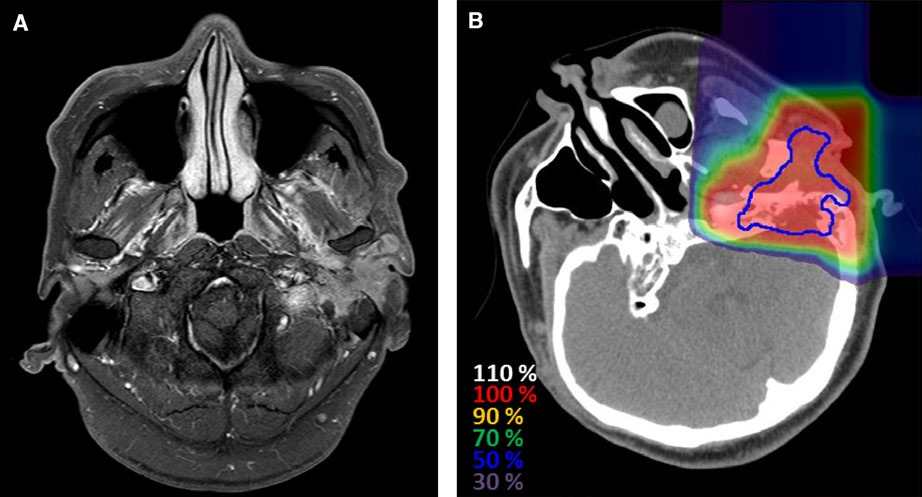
Representative case of a patient with squamous cell carcinoma of the left middle ear who was treated with carbon‐ion radiotherapy. A, Magnetic resonance imaging before carbon‐ion radiotherapy reveals a well‐enhanced left middle ear tumor B, Dose distribution. Blue lines show gross tumor volume
